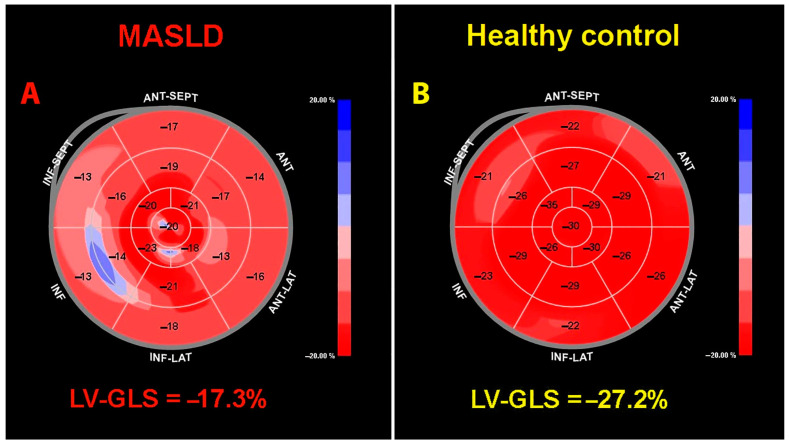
Representative examples of LV-GLS bull’s eye plot obtained by STE in an individual with MASLD (A) and in a healthy control (B). GLS, global longitudinal strain; LV, left ventricular; MASLD, metabolic dysfunction-associated steatotic liver disease; STE, speckle tracking echocardiography.
